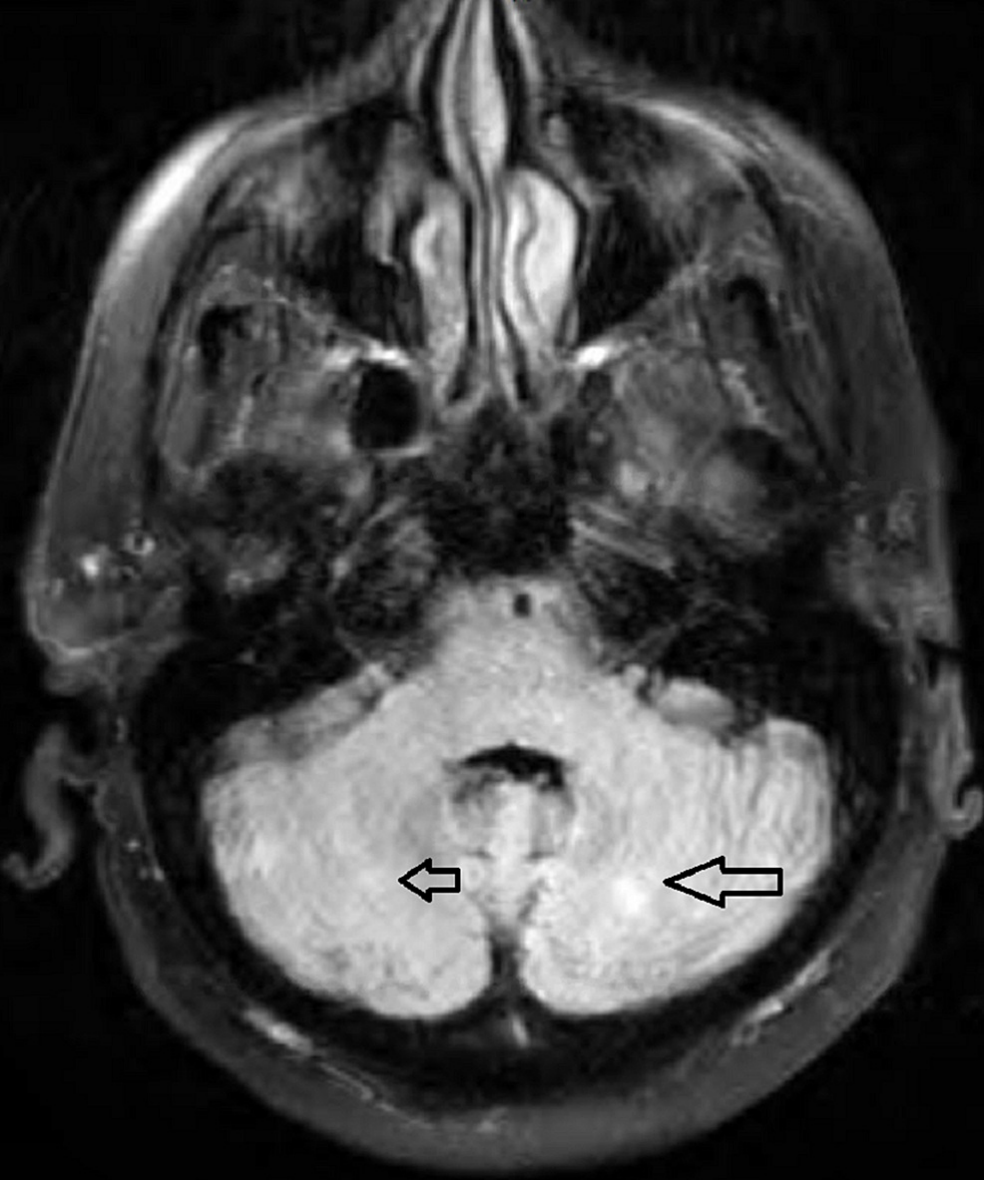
T2 flair sequence of brain MRI with and without contrast showing multiple metastatic lesions in the cerebellum (black arrows)
